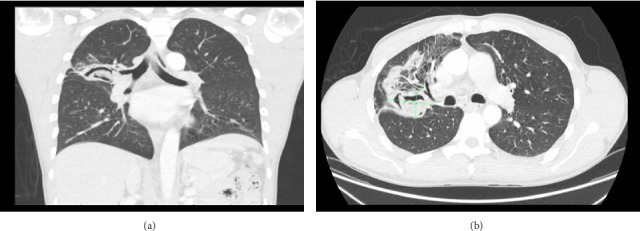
(a) Coronal section and (b) axial section of 1 month follow-up chest CT scan reveals interval decrease in size of the thick-walled cavitary lesion in the right upper lobe which now measures 5.1 × 3.2 cm and interval improvement of consolidative and ground-glass opacities in the right upper and middle lobes.
